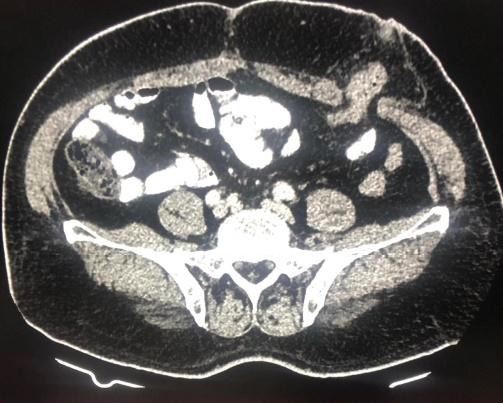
CT scan of abdomen showing end colostomy following APR.
